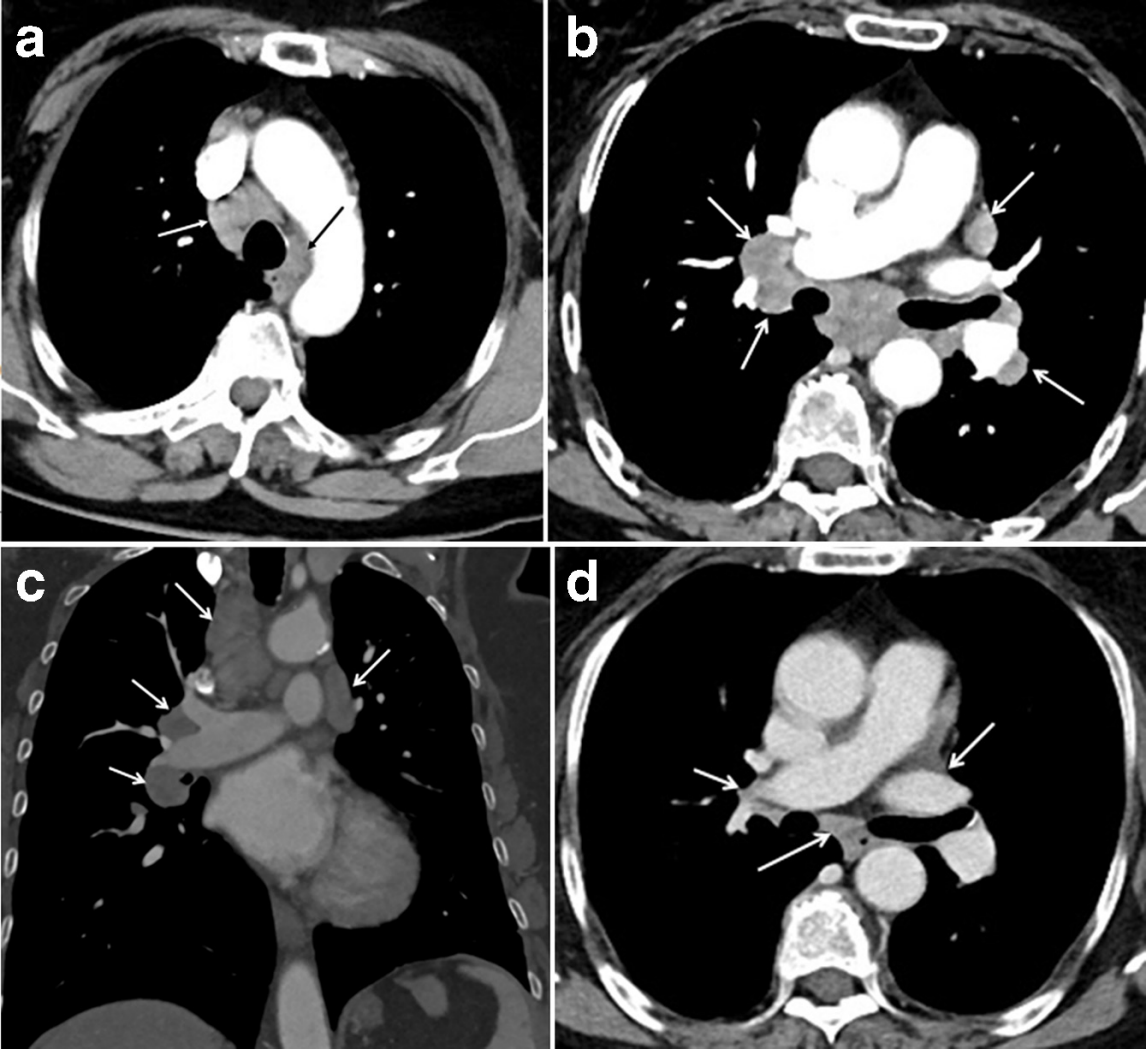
Axial (a–b) and coronal (c) thoracic contrast-enhanced CT scan shows
